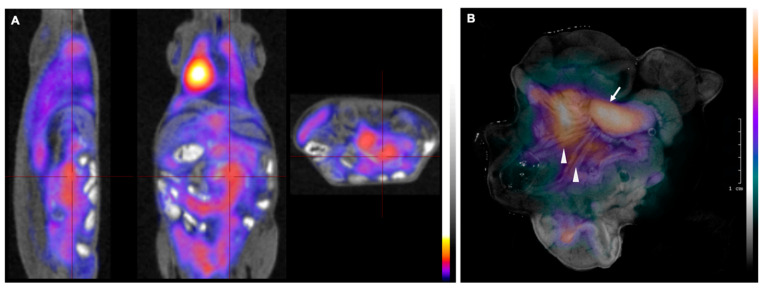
PET/MRI images of Bc-DLFL.1 lymphoma cell biodistribution in the advanced stage. PET/MRI images of Bc.DLFL1 cell biodistribution at advanced lymphoma stage after intraperitoneal tumor inoculation detected by intravenous FDG injection (administered 1 h earlier) show several mesenteric lymph nodes accumulating (A). After subsequent removal, the abdominal organs were subjected to CLI ex vivo. Panel B shows superimposed white and Cerenkov light pictures. The arrow indicates extensive FDG accumulation in the mesenteric lymph node conglomerates (arrow). In addition to mesenteric lymph nodes, CLI also detected the lymphoma expansion within mesenterial fatty streaks (arrowheads) (B).
